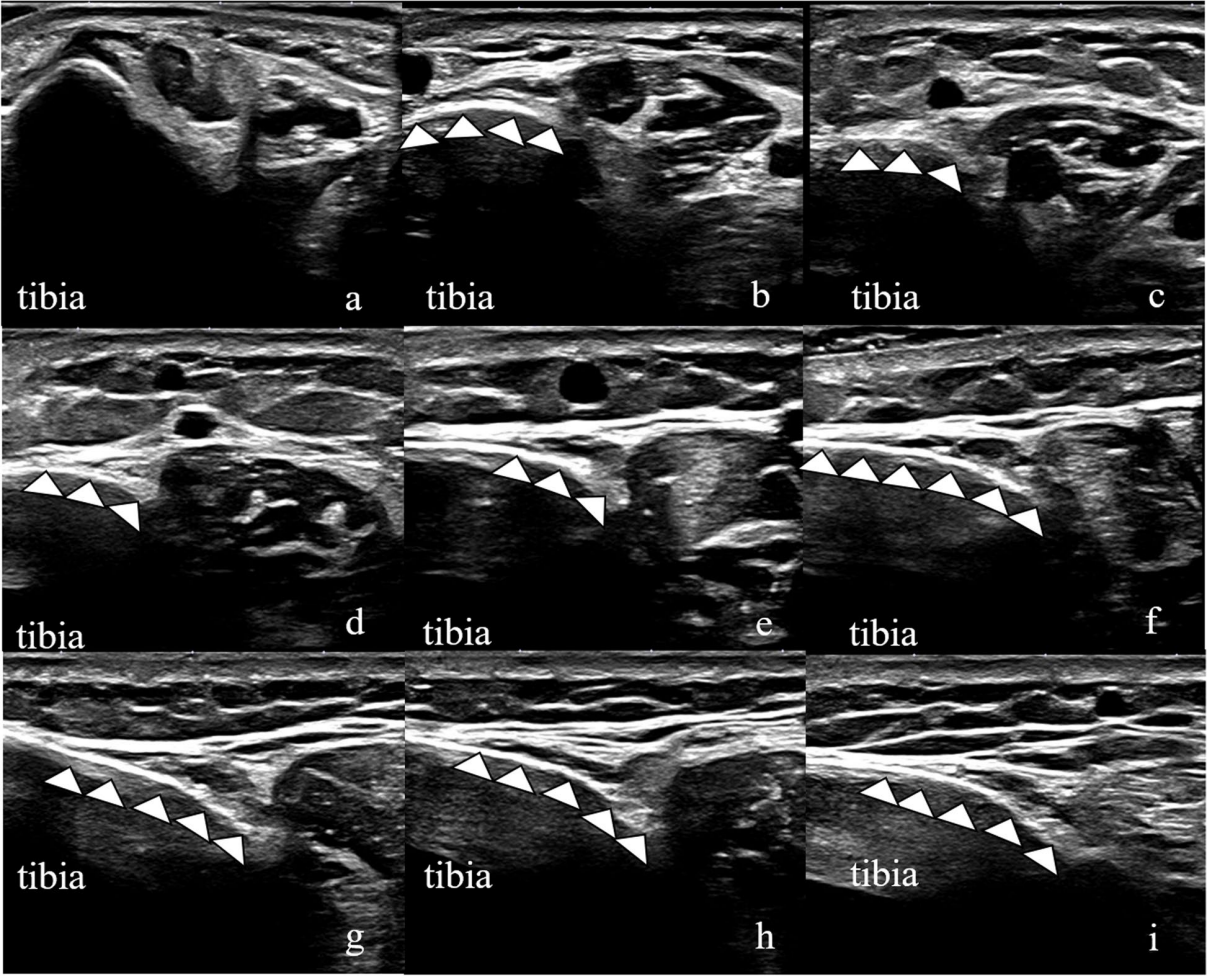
A 22-year-old man. Ultrasound images of the transverse axis of the medial calf. The adipose tissue is visualised with high echogenicity (white arrows). Images acquired at a: 3 cm; b: 6 cm; c: 9 cm; d: 12 cm; e: 15 cm; f: 18 cm; g: 21 cm; h: 24 cm; and i: 27 cm from the distal tip of the medial malleolus. These images are from the same participant as in Fig. 4
